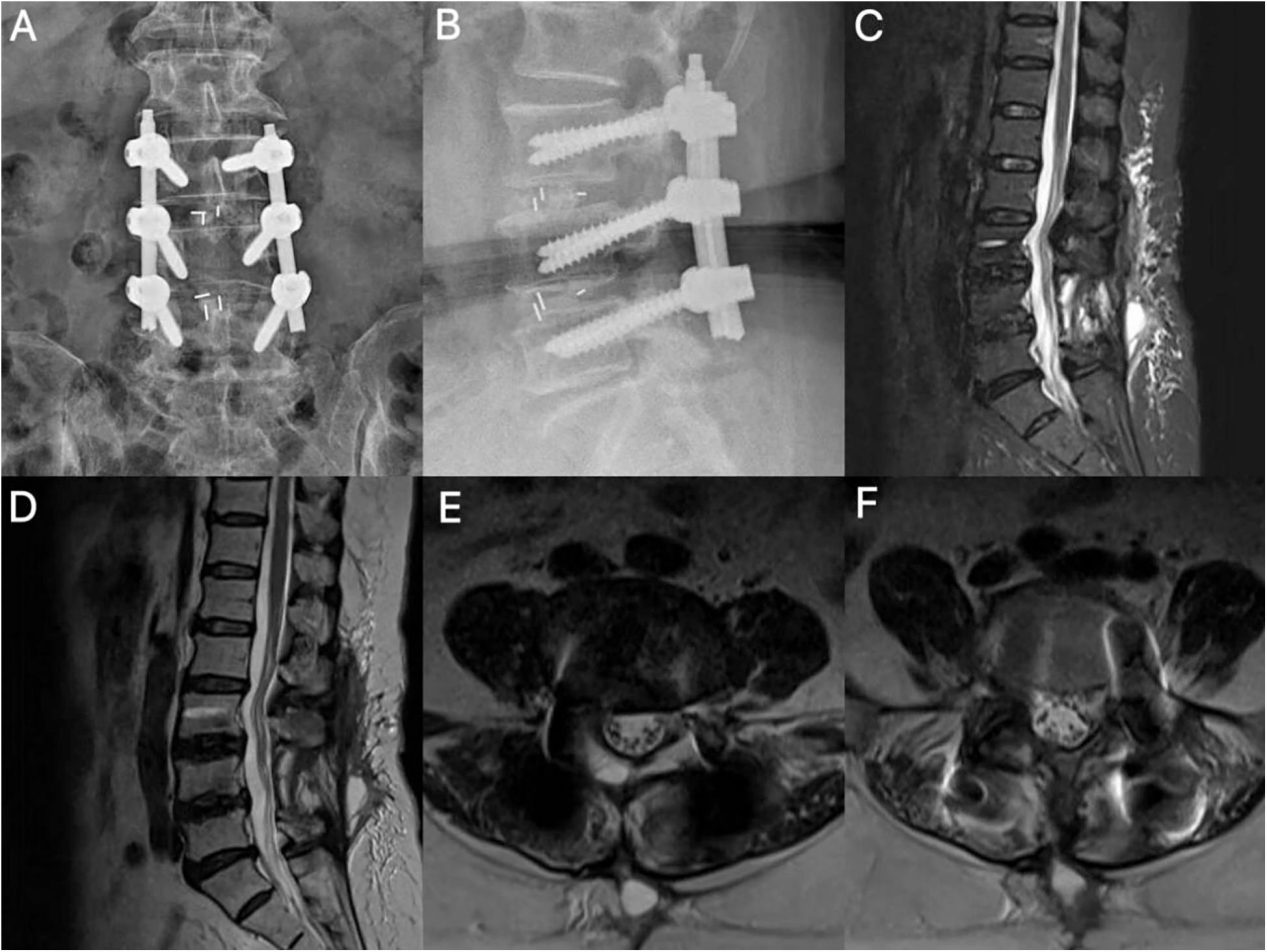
Postoperative imaging. (A,B) Lumbar radiographs demonstrate accurate positioning of L3–L5 pedicle screws and optimal interbody cage placement. (C,D) Sagittal MRI reveals spinal canal expansion and localized epidural fibrosis. (E,F) Segments L3–4 and L4–5 are shown with no space-occupying lesions; note the ventral cauda equina apposed to the dural sac in a hypertension state with a positive sedimentation sign (+).
